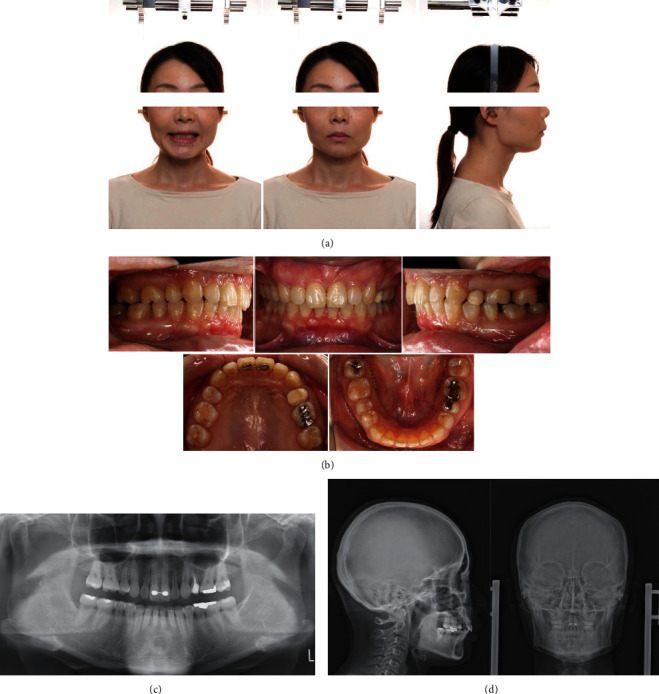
Posttreatment records. (a) Extraoral photographs. (b) Intraoral photographs. (c) Orthopantomograms. (d) Posteroanterior and lateral cephalograms.
